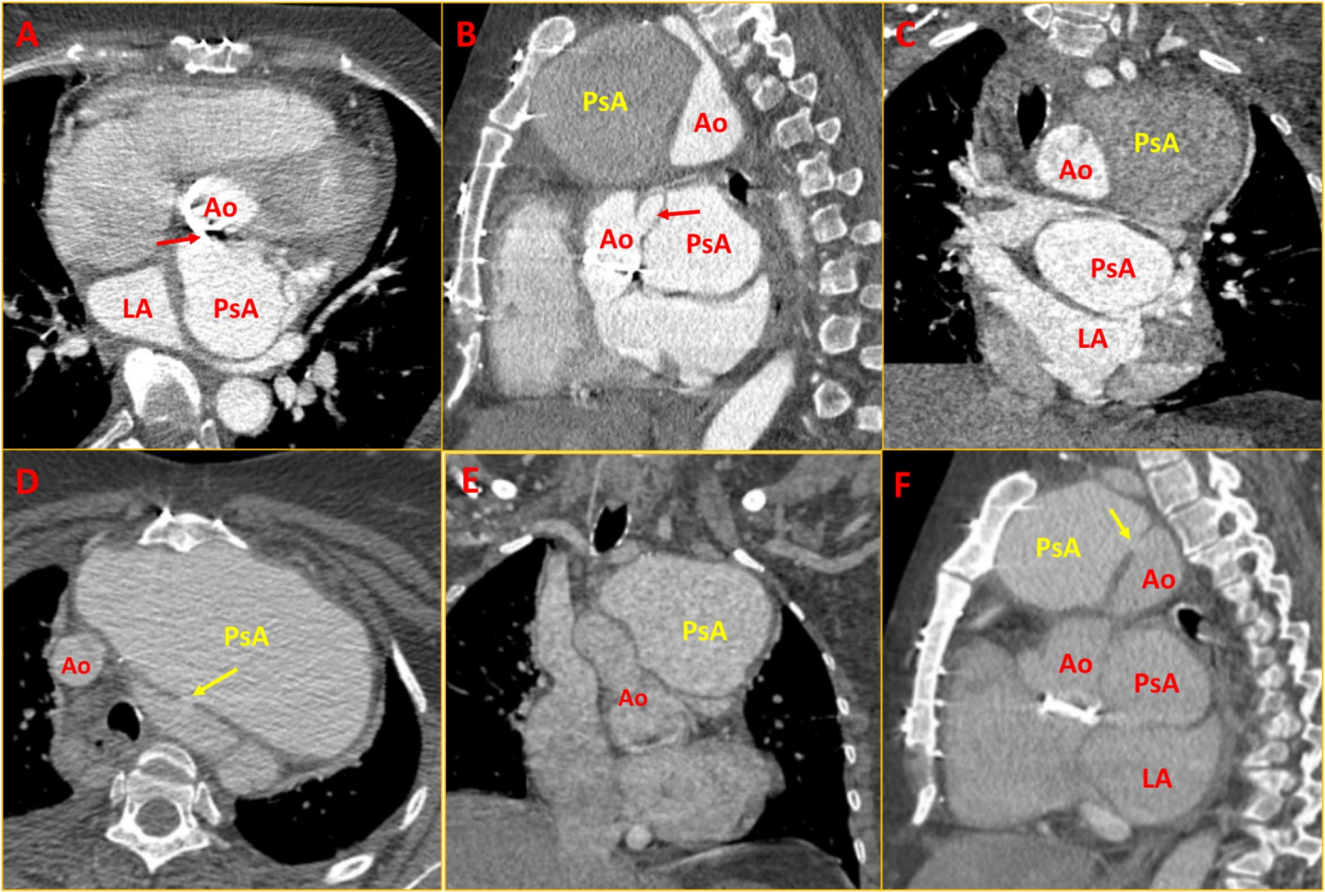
Cardiac CT. A Axial CT aortogram showing large PsA arising from aortic root just above the prosthetic valve, compressing the LA (arrow: neck of PsA); B Sagittal CT aortogram showing 2 PsAs, one posteriorly from aortic root with good contrast opacification in early phase, second anteriorly from ascending Ao abutting the sternum (note the left coronary artery dilatation [arrow] and LA compression by aortic root PsA); C Coronal CT aortogram showing the special relationship of the PsAs with Ao and LA. (D-F) Delayed phase of aortogram in axial (D), coronal (E), and sagittal (F) view showing the above findings (arrow: neck of PsA). CT = computed tomography; PsA = pseudoaneurysm (yellow: at ascending aorta, red: at aortic root); Ao = aorta; LA = left atrium
